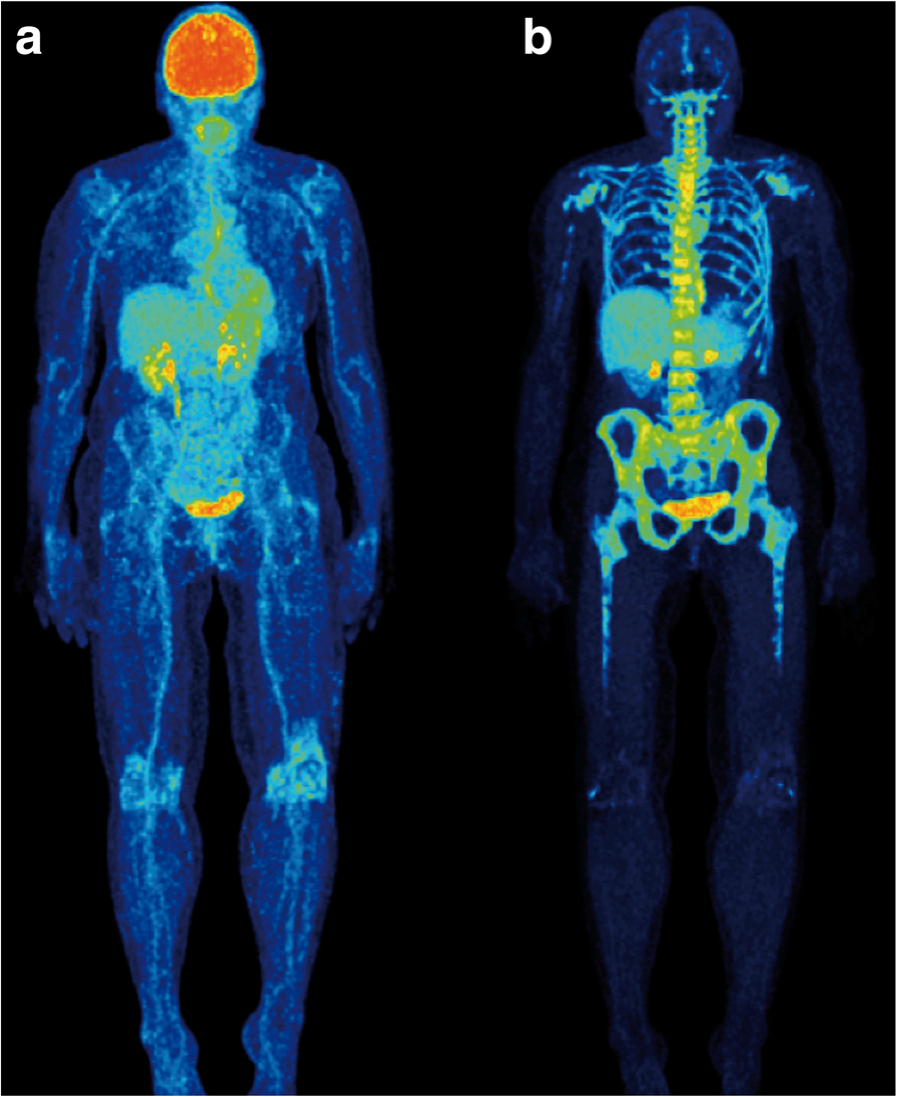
Maximum intensity projection (MIP) ^18^F-FDG PET/CT (a) and ^18^F-FLT PET/CT (b) of a 76-year-old female SMM patient. No focal, myeloma-indicative lesions detected with either tracer. Normal ^18^F-FLT distribution is characterized by high background activity by the bone marrow compartment (e.g., vertebral column, pelvic skeleton, proximal femora) reflecting the high proliferative activity of hematopoietic cells
